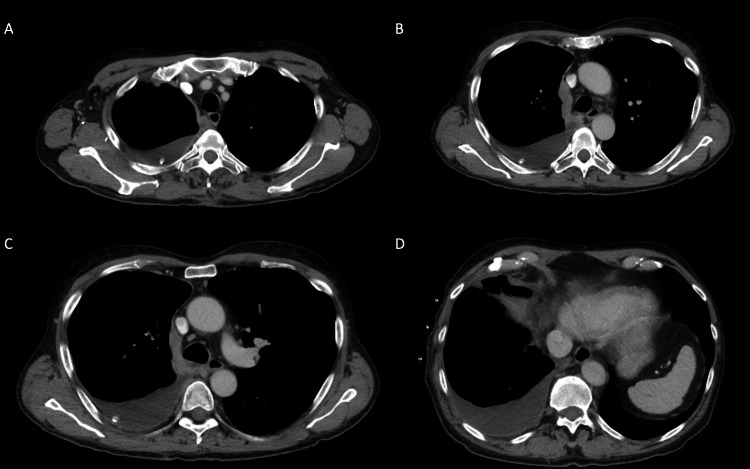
CT Chest at time of diagnosis, after pleural effusion drainage. A) Pleural-based disease along superior aspect of mediastinum measuring thickness of 12 mm. B) Pleural-based conglomerate abutting lateral superior mediastinum, measuring up to 17 mm in thickness. C) Pleural-based plaque extending down mediastinum. D) Pleural effusion at right lung base.
